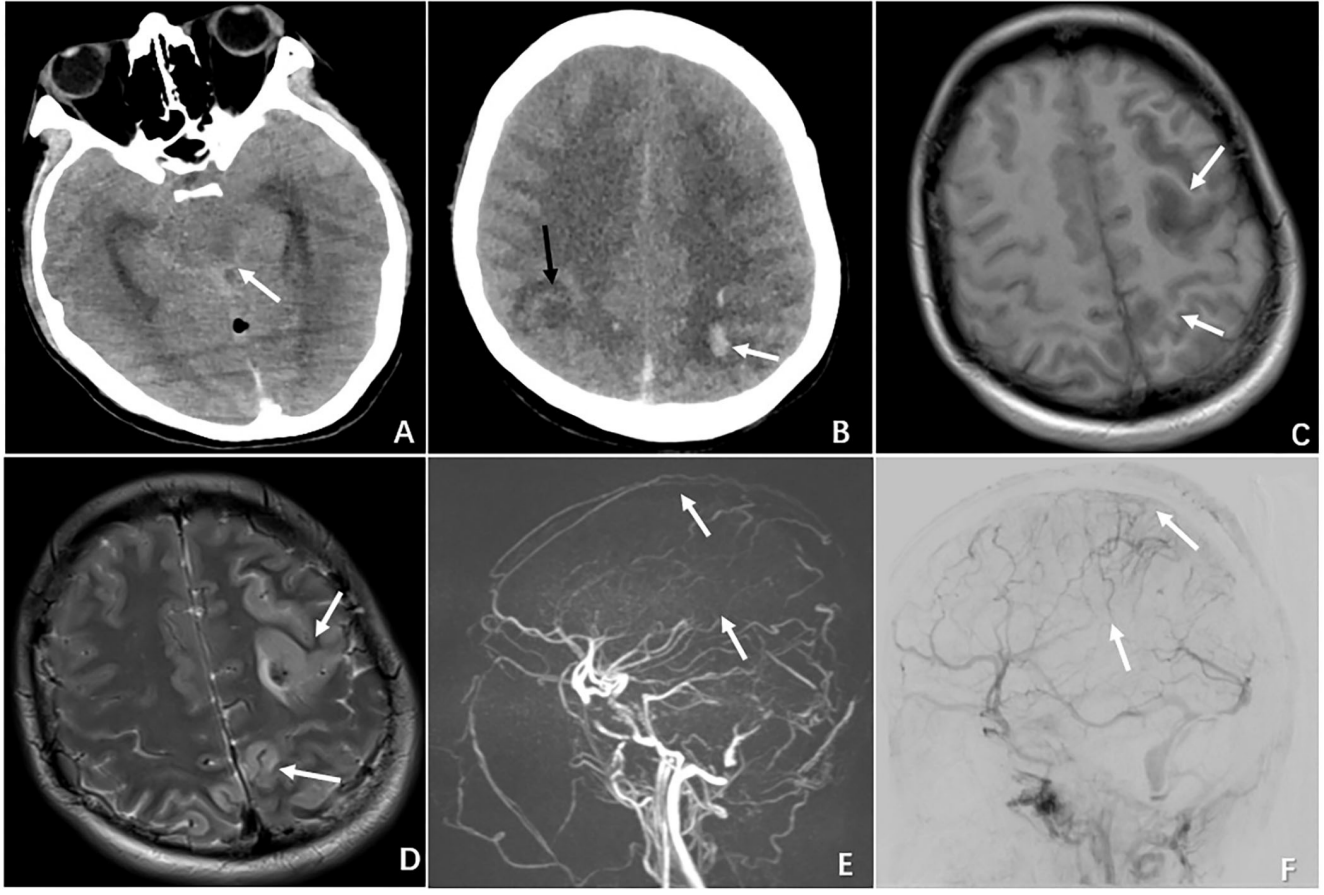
Radiographic features of CVT in the case of N6 who presented with severe neurological deterioration. (A) Non-contrast CT demonstrating brain swelling and ambient cistern compression (white arrow). (B) CT demonstrating multiple ischemic infarctions (black arrow) with a hemorrhagic component (white arrow). (C) MRI showing a left hyperintense lesion with a surrounding hypointense area on T1-weighted MRI. (D) MRI demonstrating the same hypointense lesion with a surrounding large hyperintense area on T2-weighted MRI. (E) MRV showing complete occlusion of the superior sagittal sinus (superior arrow) and inferior sagittal sinus (inferior arrow). (F) DSA demonstrating occlusion of the superior sagittal sinus (superior arrow) and inferior sagittal sinus (inferior arrow).
